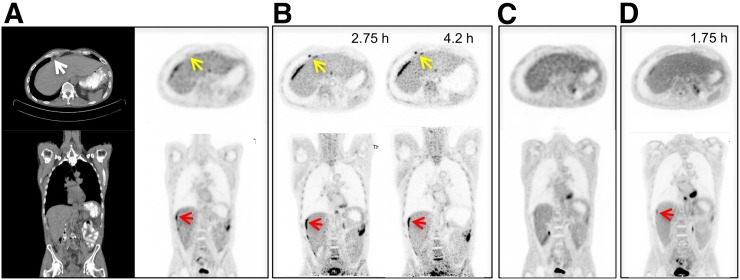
Clinical ^18^F-FDG PET/CT images (transverse and coronal) from subject 5, with metastatic colon cancer, acquired with standard clinical protocol. (B) PennPET image acquired 2.75 and 4.2 h after injection (10-min scans). Matched coronal and transverse slices are shown. Red arrows denote perihepatic disease; yellow arrows denote epiphrenic lymph node. (C) Follow-up clinical scan at 3 mo (subject 10). (D) Corresponding PennPET image (20-min scan) demonstrating improvement in perihepatic disease and epiphrenic lymph node.
